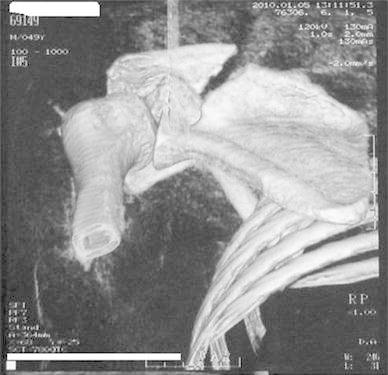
Axial computed tomography (three dimensional)
